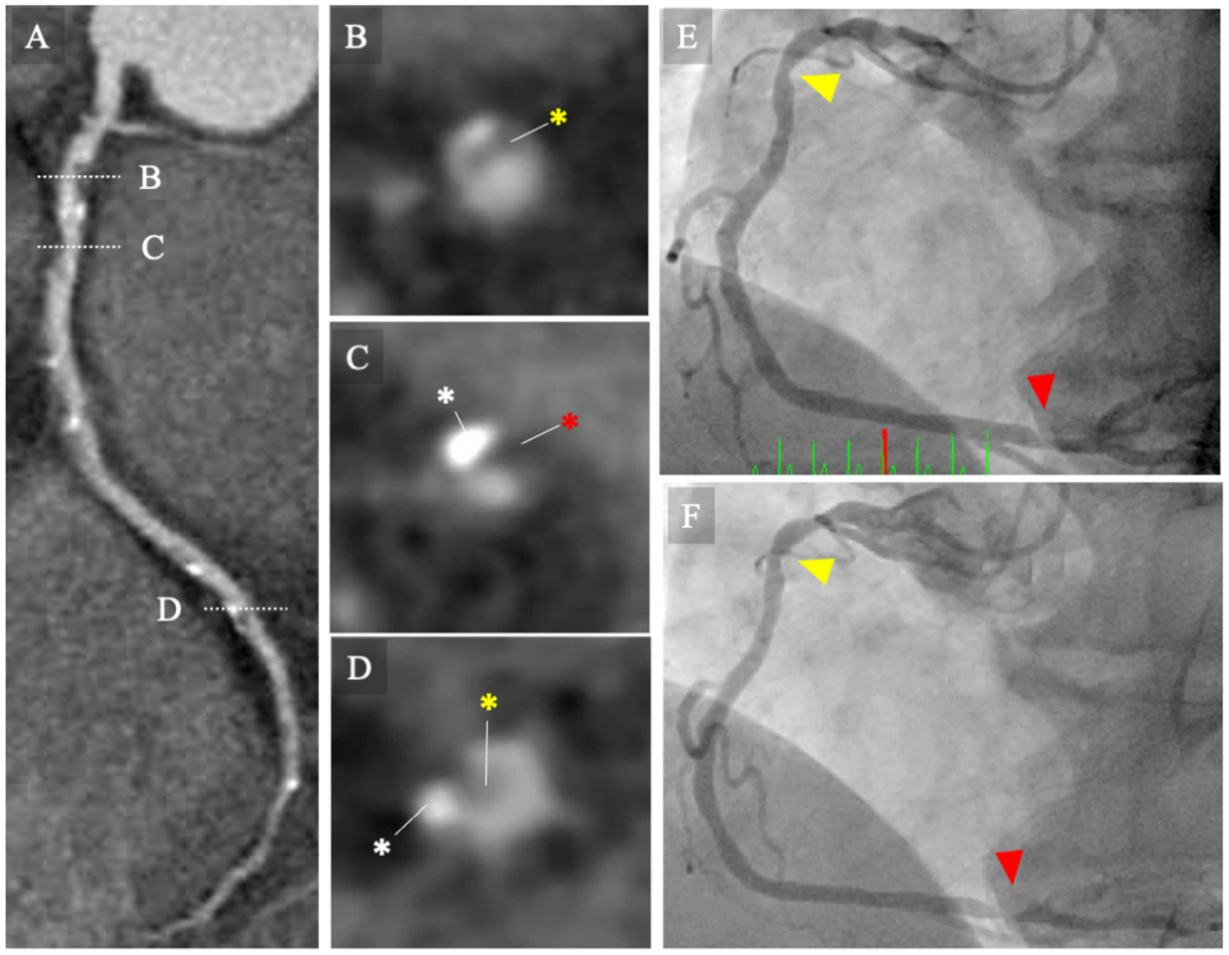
CCTA images and invasive coronary angiography. (A–D) CCTA images of a patient with non-obstructive CAD and increased low-attenuation plaque (Q4) who developed to unstable angina requiring urgent coronary revascularization. The MPR image of the baseline CCTA image for right coronary artery (RCA) showing intermediate stenosis severity with low-attenuation coronary plaque (Q4). (B–D) Cross-sectional images of coronary lesions (white broken bars). (B) Mild stenosis with non-calcified plaque (yellow asterisk). (C) Intermediate stenosis with calcified plaque (white asterisk) and low-attenuation plaque (red asterisk). (D) Intermediate stenosis with calcified and non-calcified plaques. (E) Invasive coronary angiography performed at 12 days following the baseline CCTA examination. Invasive coronary angiography (ICA) image shows intermediate stenosis of the proximal (yellow arrowhead) and distal portions of the RCA (red arrowhead). The patient was managed with conservative strategy, including statins. (F) The patient presented with unstable angina and underwent emergency ICA at 1.5 years following the baseline CCTA examination. The ICA image revealed progression of the coronary lesions (red arrowheads). CCTA, coronary computed tomography angiography; ICA, invasive coronary angiography; MPR, multi-planar reconstruction; RCA, right coronary artery.
